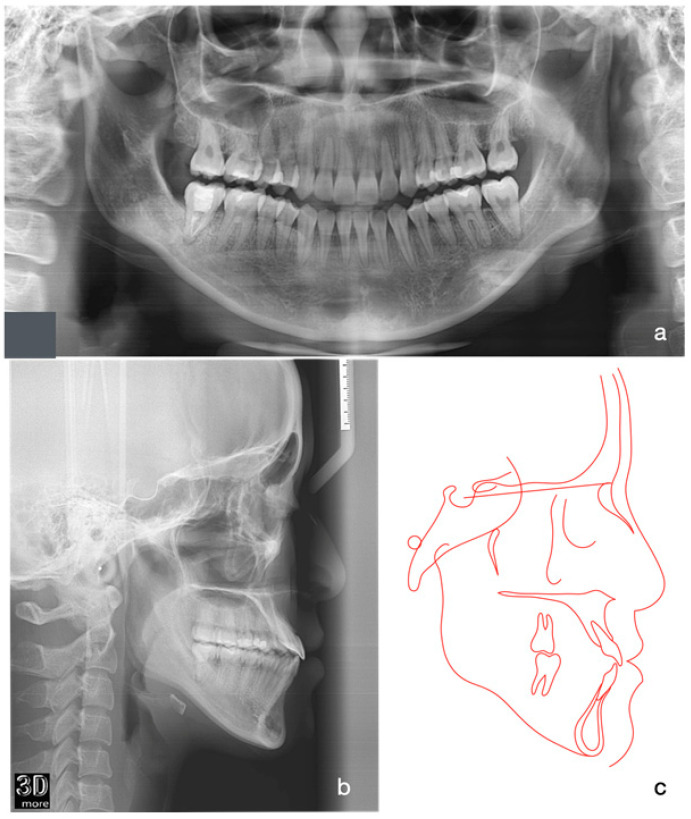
Final orthopantomographic (OPG) and lateral cephalometric radiographs and tracing. Good root parallelism was achieved, with no significant root resorption observed (a). Lateral cephalometric radiographs and tracings demonstrate a skeletal Class I relationship with reduced chin projection. Both the upper and lower incisors show proper inclination (b,c).
